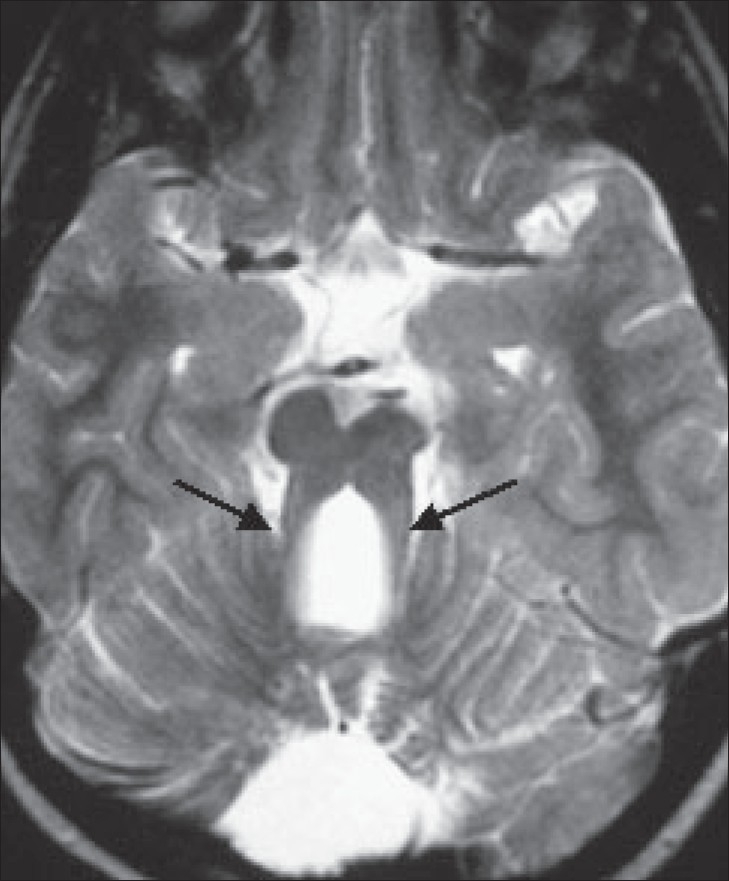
Axial T2W MRI of the brain shows the typical ‘molar-tooth’ configuration of the superior cerebellar peduncles, with a hypoplastic cerebellar vermis
